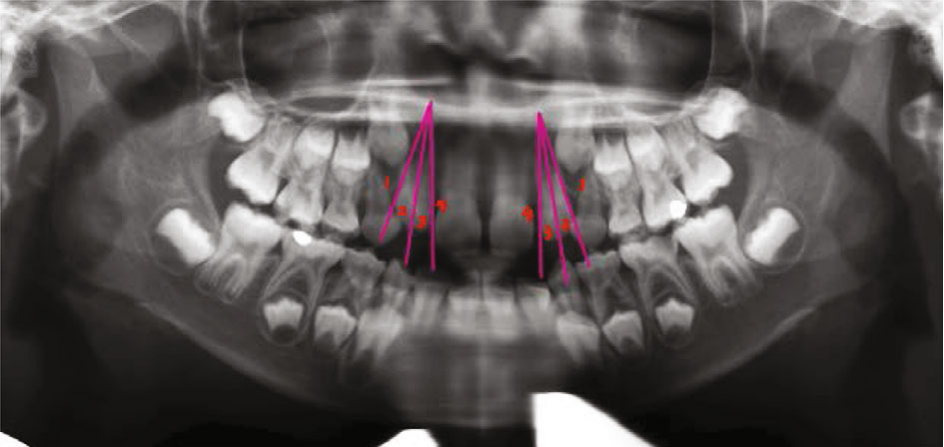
Showing parameter 3 traced on panoramic radiograph.
